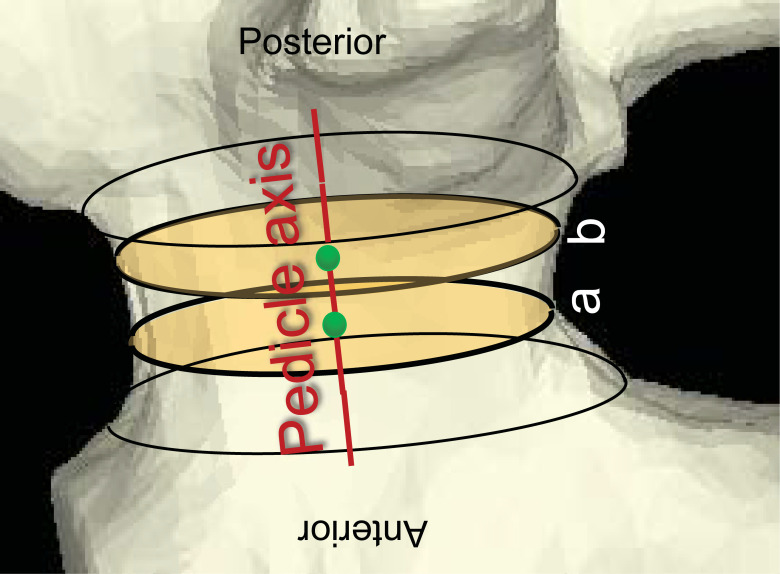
Determination of the pedicle axis at the 3D CT model. The green spheres are centroids at cross sections located at the anterior one-third and the posterior one-third of the pedicle. The yellow ellipses show the cross sections located at the anterior one-third and the posterior one-third of the pedicle. The red line is axis of the pedicle.
